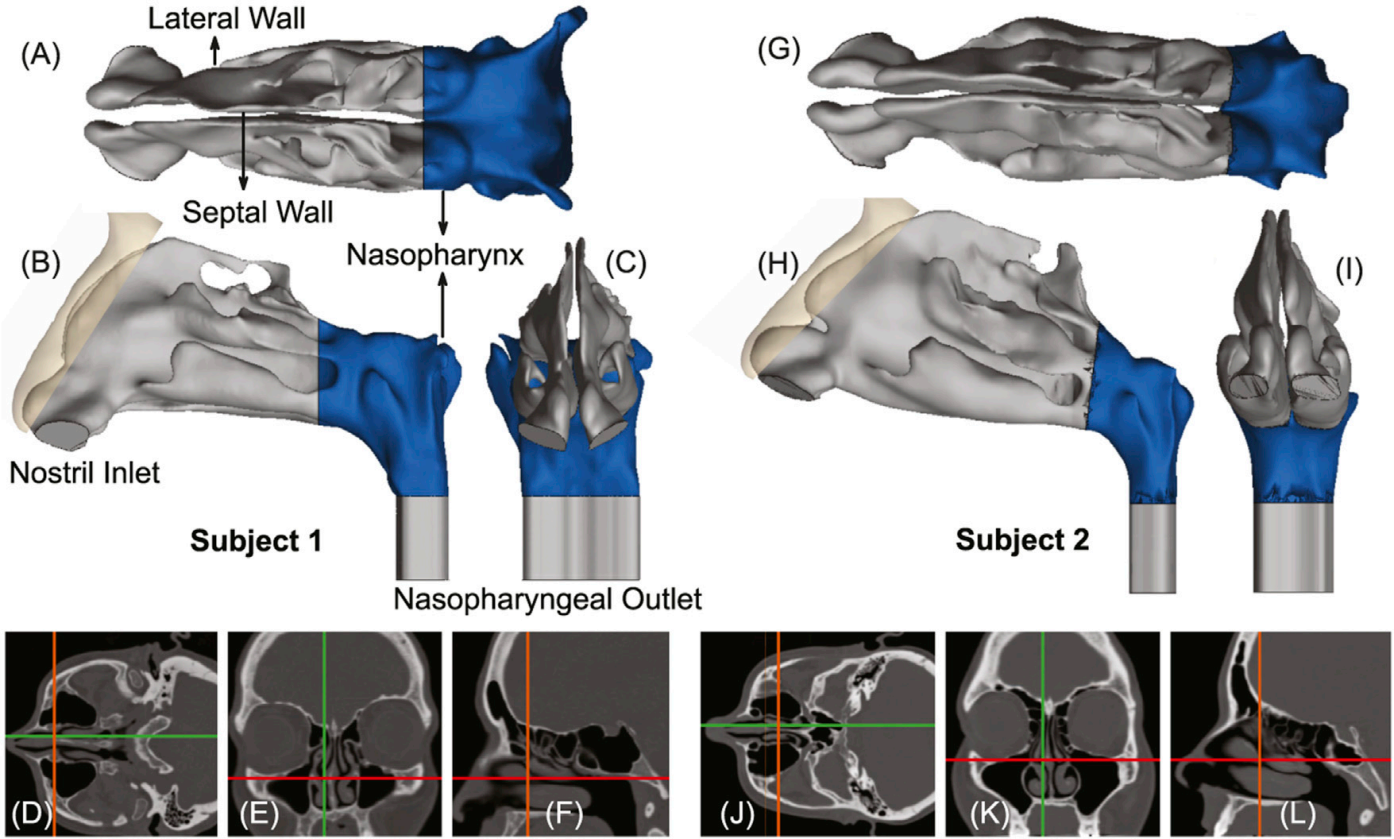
Panels (A–C), respectively, show the axial, sagittal, and coronal views of the computed tomography (CT) based upper airway reconstruction in Subject 1. Panels (D–F) depict representative CT slices for the same subject. Therein, the green lines in (D, E) correspond to the location of the sagittal section shown in (F); the orange lines in (D, F) correspond to the location of the coronal section shown in (E); the red lines in (E, F) correspond to the location of the axial section shown in (D). Panels (G–I) respectively show the axial, sagittal, and coronal views of the CT-based upper airway reconstruction in Subject 2. Panels (J–L) depict representative CT slices for the same subject. Therein, the green lines in (J, K) correspond to the location of the sagittal section shown in (L); the orange lines in (J, L) correspond to the location of the coronal section shown in (K); the red lines in (K, L) correspond to the location of the axial section shown in (J). The nasopharynx has been marked in blue in panels (A–C) and (G–I).
